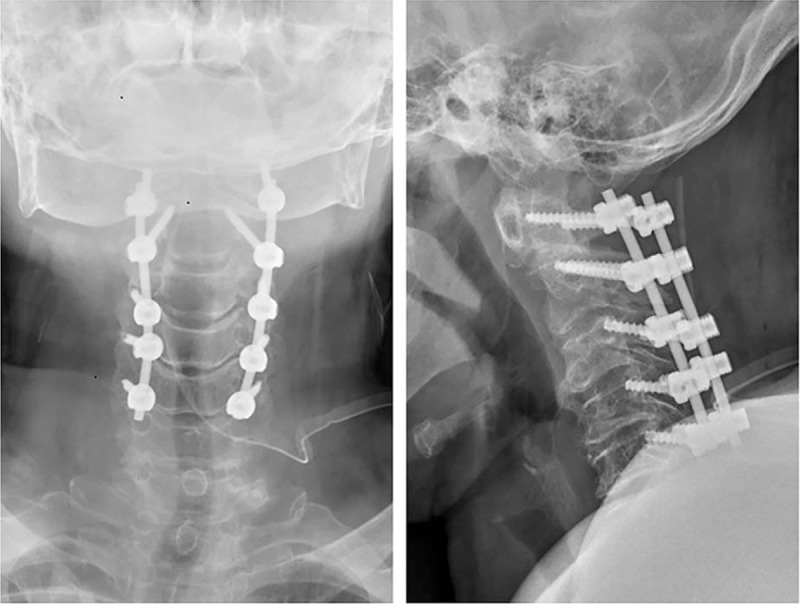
The postoperative x-ray showed good location of internal fixation device.
